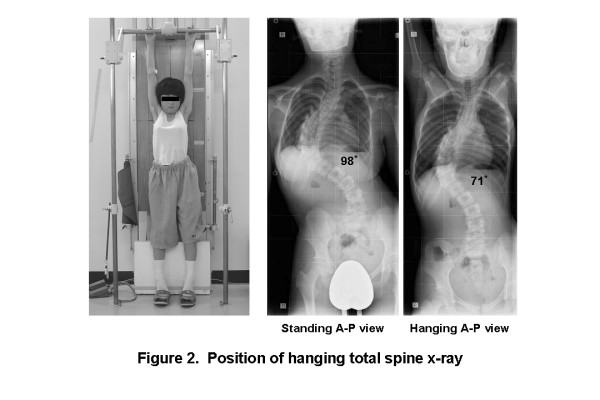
Position of taking hanging total spine x-ray. Hanging total spine x-ray was taken in position that the patient is hanging onto the bar, stretching the back, and touching the toes lightly to the floor not to sway the body. Patients were instructed making a great effort to stretch their back as much as possible.
